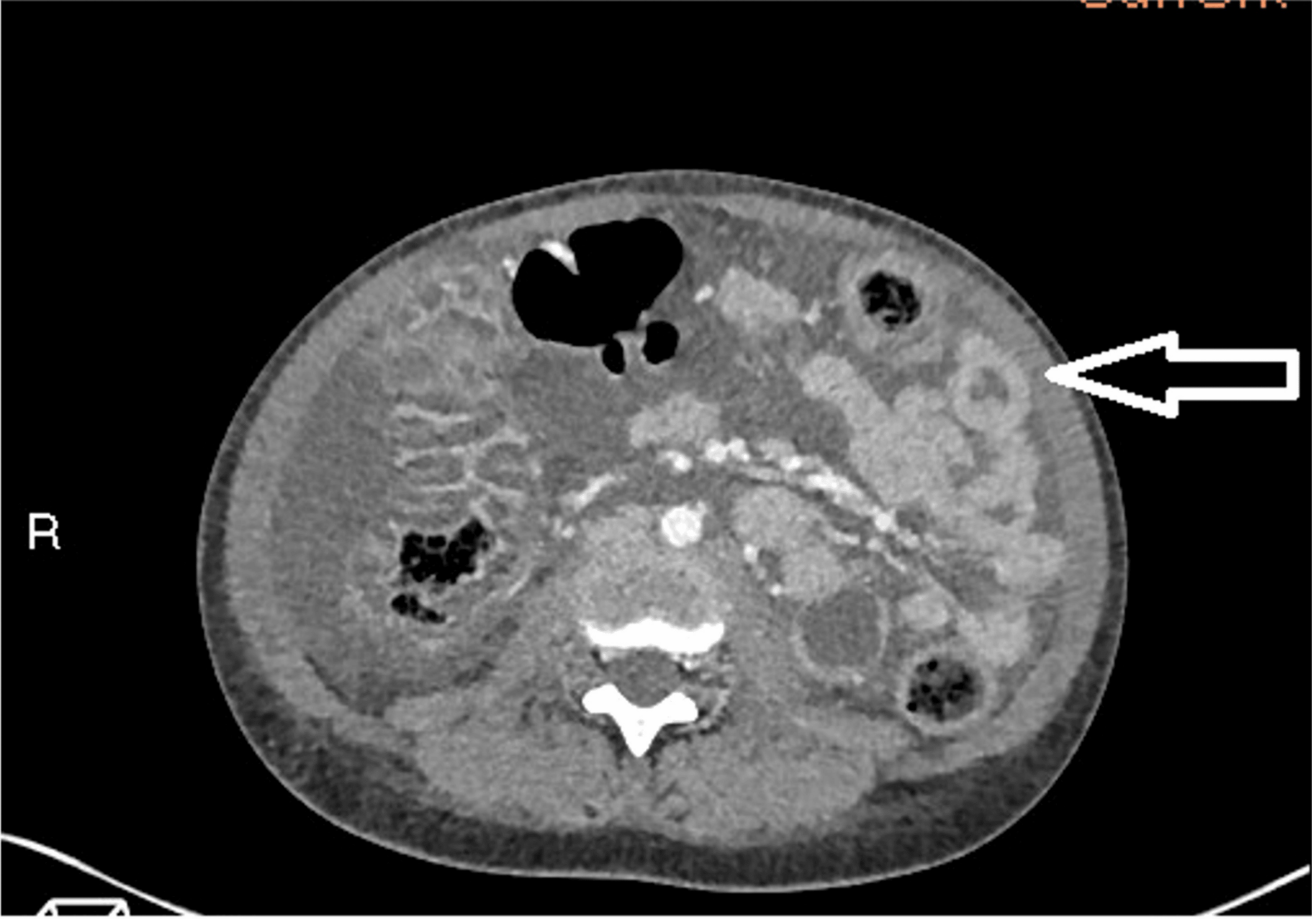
Contrast-enhanced CT of the abdomen and pelvis in the axial plane demonstrating the “target sign” Axial cross-sectional CT image of the abdomen and pelvis showing inflammation within the bowel loops. The jejunum (arrow) shows the typical “target sign”, as described above. Lupus has a predilection for jejunal inflammation. The target sign may also be observed in other active inflammatory conditions such as inflammatory bowel disease, ischaemic bowel and infectious colitis. In lupus, a lack of other typical IBD or neoplastic findings on imaging, plus serological evidence of SLE activity, can guide the correct diagnosis.
